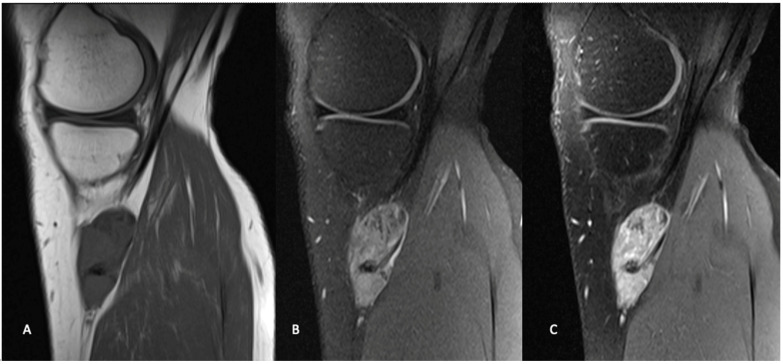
Sagittal MR images in the same patient demonstrate a periarticular soft tissue mass situated posteromedial to the proximal tibia, in close relation to the pes anserine tendons. The mass demonstrates intermediate signal on T1 weighted images (A), and heterogeneously high signal on T2 weighted fat-saturated images (B). T1 weighted fat-saturated after gadolinium administration (C), demonstrates heterogeneously avid enhancement. Known areas of calcification are low signal on all sequences (arrow).
